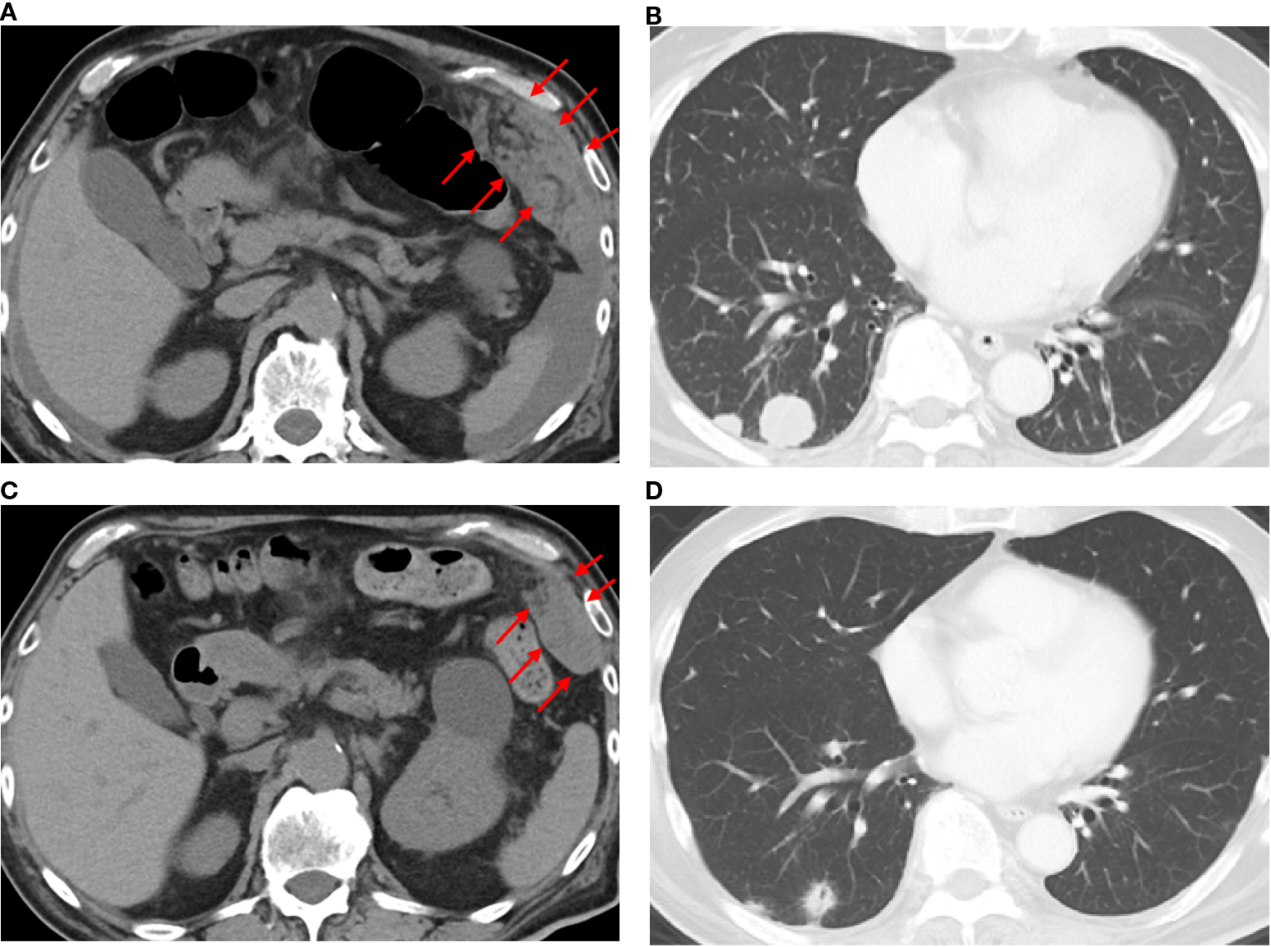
Computed tomography findings before and after the administration of zolbetuximab-based chemotherapy. CT images before treatment (a, b) and 2 months after zolbetuximab-based chemotherapy (c, d). Red arrows indicate peritoneal disseminated nodules.
